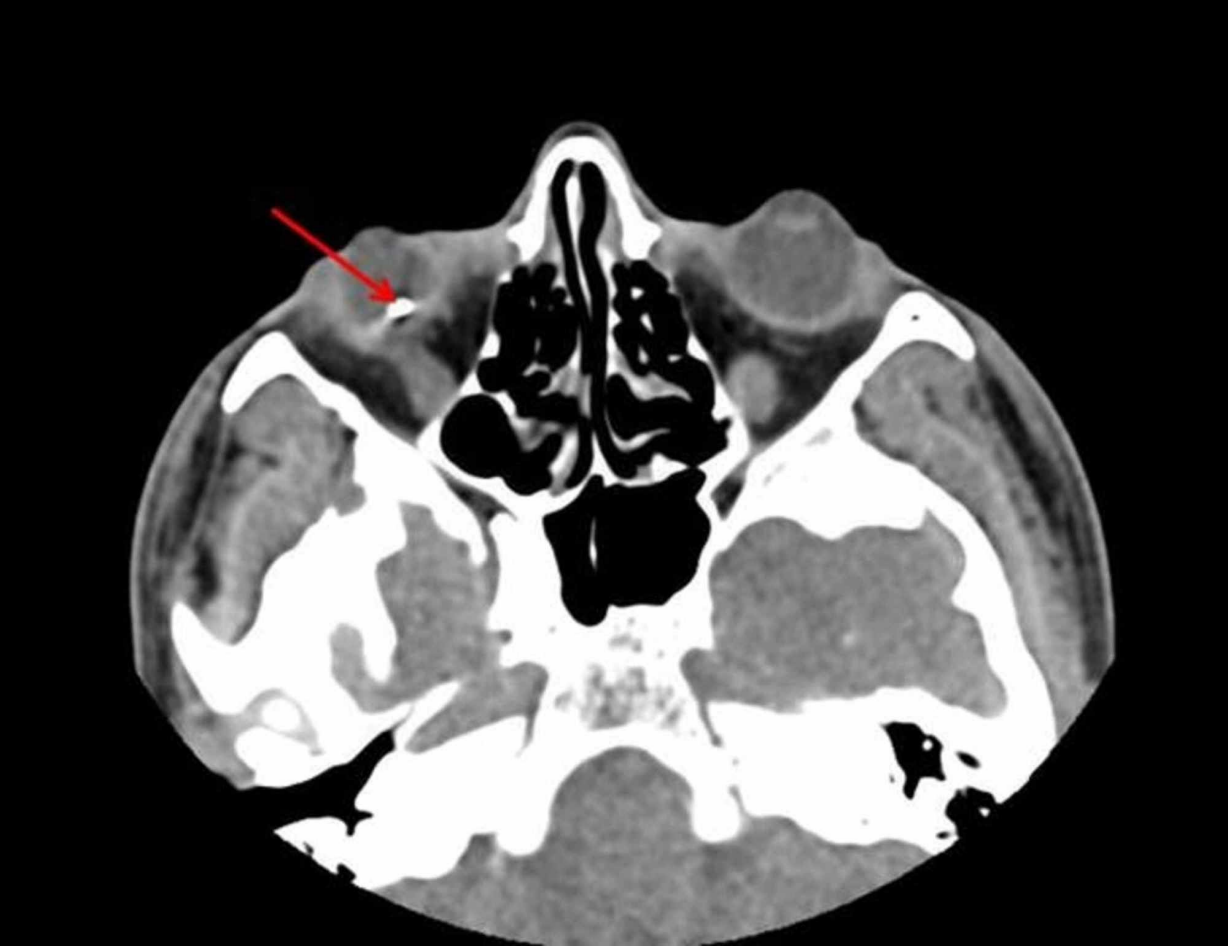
CT scan without contrast of the orbits confirming the presence of a retained intraocular foreign body in the right eye. Red arrow points to the presence of an intraocular foreign body in the right eye.
